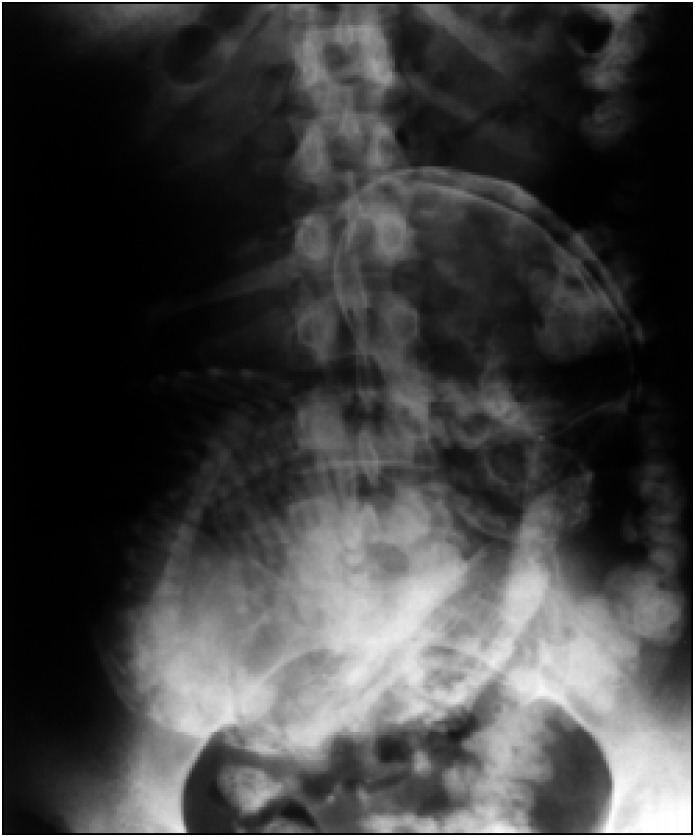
Calcified Abdominal Pregnancy - Abdominal X-ray.
